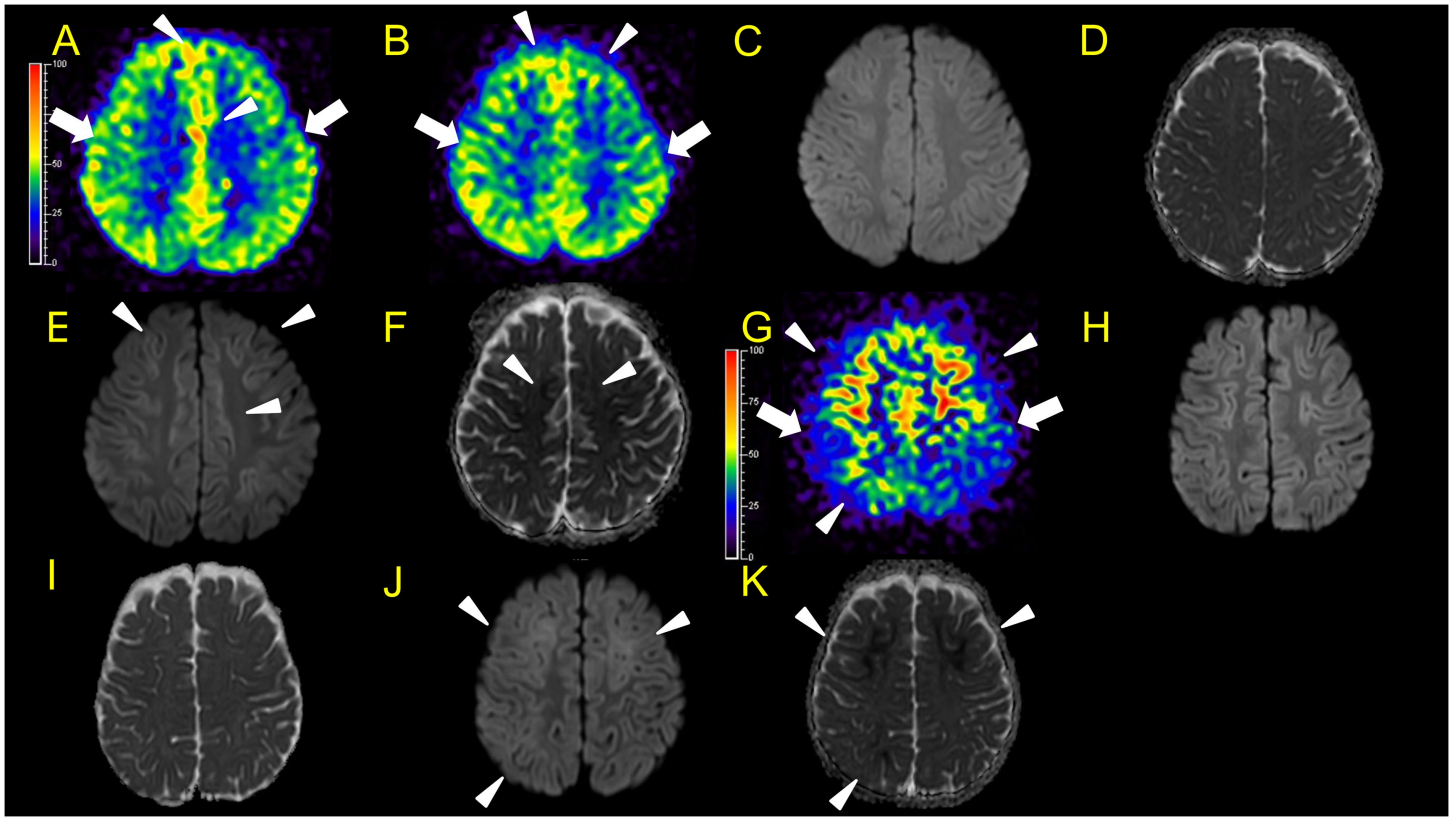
Neuroimaging findings. Colour bars for ASL indicate signal intensities, not absolute perfusion values. Patient 5 (A–F): A–D, ASL (A,B, post-labelling delay 1,500 ms), DWI, ADC at 42 h after ESs. ASL revealed hyperperfusion in the bilateral frontal regions (A,B: arrowheads) compared to perirolandic regions (arrows), while DWI or ADC showed no abnormalities (C,D). Although there is the possibility that hypoperfusion areas exist in the left lateral frontal lobe, we were unable to evaluate the signal ratio between the cortical abnormal perfusion region and the perirolandic region due to the technical reason mentioned in the materials and methods section. (E,F) DWI and ADC on day 10. Hyperperfusion areas in bilateral frontal lobes in ASL at 42 h from ESs coincide with BTA on day 10 (E,F: arrowheads). Patient 6 (G–K), G–I, ASL (post-labelling delay 2000 ms), DWI, ADC at 82 h after ESs. (J,K) DWI and ADC at 103 h. Hyperperfusion areas in bilateral frontal lobes and right parietal lobe in ASL at 82 h after ESs (G: arrowheads) compared to the perirolandic region (arrows), while DWI or ADC showed no abnormalities, which coincide with BTA at 103 h (J,K: arrowheads).
